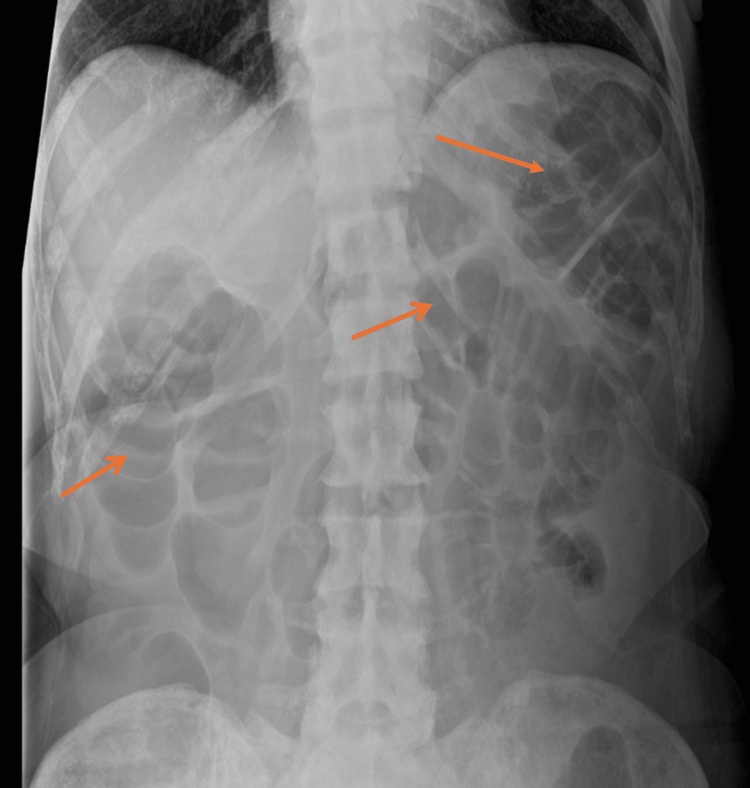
Abdominal X-ray showing prominent bowel loops without significant dilatation (arrows).
